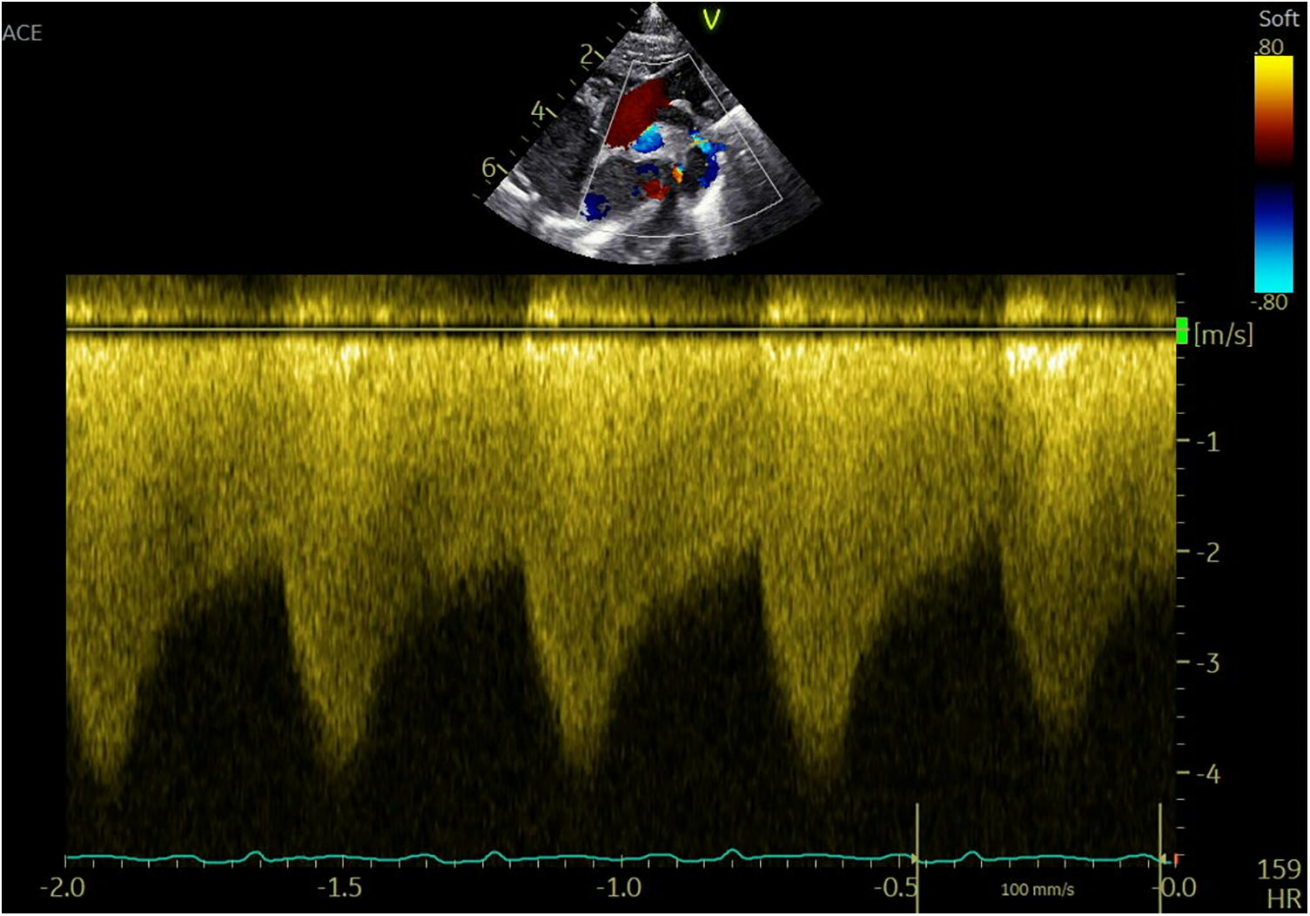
Color Doppler demonstrating turbulent flow at the coarctation site (peak velocity: 4.1 m/s).
